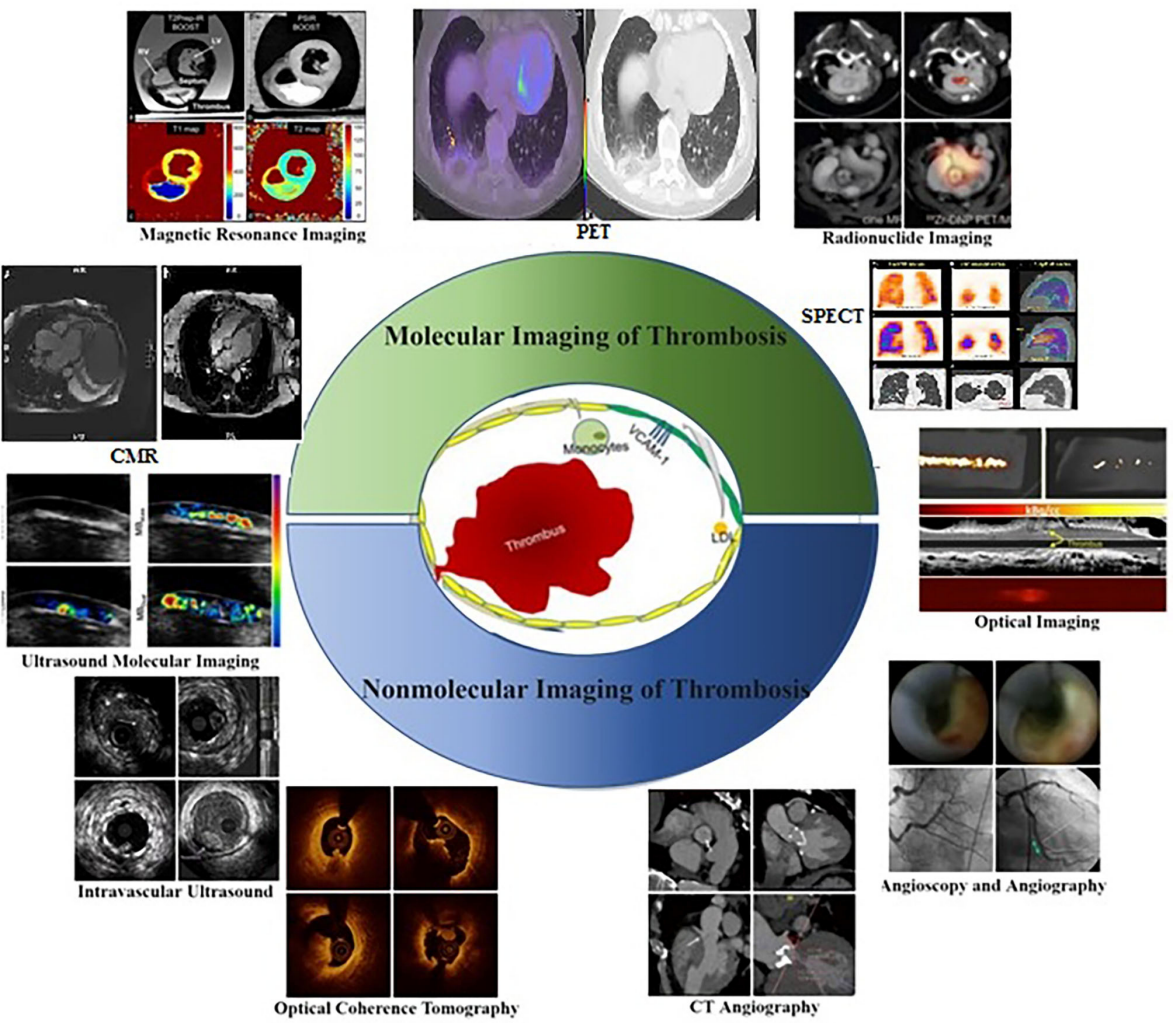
Molecular and Non-molecular thrombosis imaging strategies in atherosclerosis patients for assessing pathophysiological features related to early thrombosis at the molecular and cellular level (13), including ultrasound molecular imaging (6), magnetic resonance imaging (7), radionuclide imaging (14), optical imaging (15), intravascular ultrasound, optical coherence tomography, CT angiography (16), angioscopy and angiography (17).
